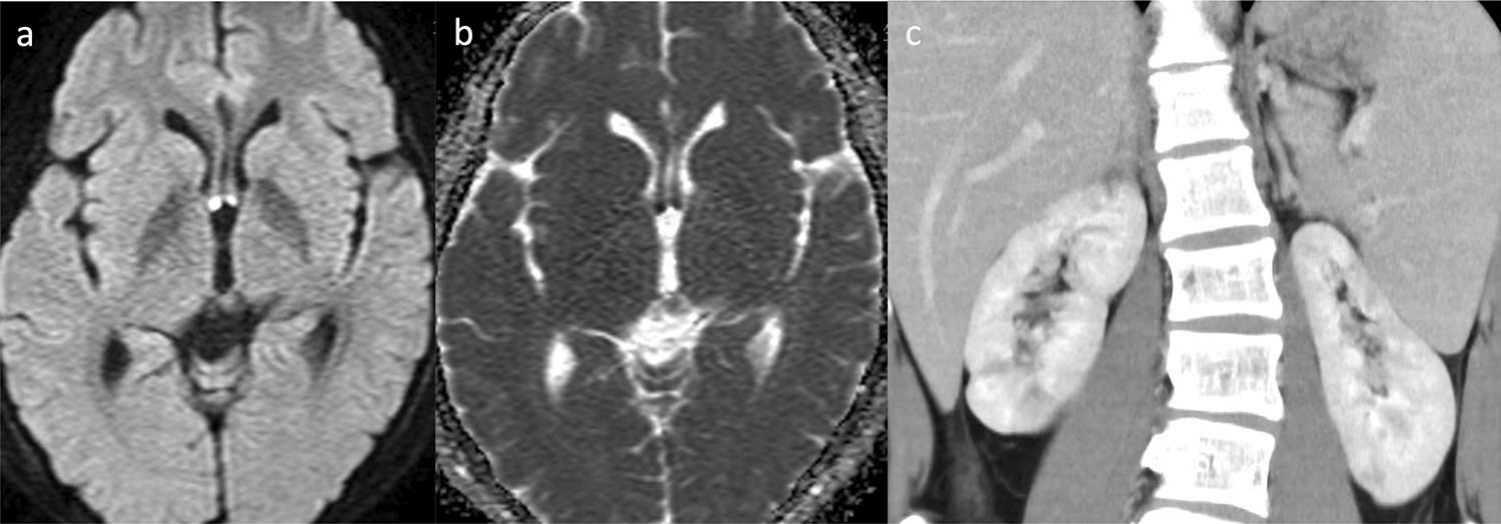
Deficiency of adenosine deaminase type 2 (DADA2) in a male patient aged 18 years who received the diagnosis of DADA2 at the same time as his older brother (this patient has already been reported by Nihira et al. “P3” corresponds to this case). [142] Axial DWI (a) and ADC map (b) exhibited diffusion restriction in the bilateral column of the fornix, leading to the diagnosis of fornix infarction. The patient experienced memory loss spanning 2–3 days prior to MRI imaging. MR angiography found no stenosis in the major branches (not shown). Contrast-enhanced computed tomography (c) demonstrated impaired renal cortical enhancement and irregularities suggestive of a previous renal infarction. Additionally, splenomegaly was observed
